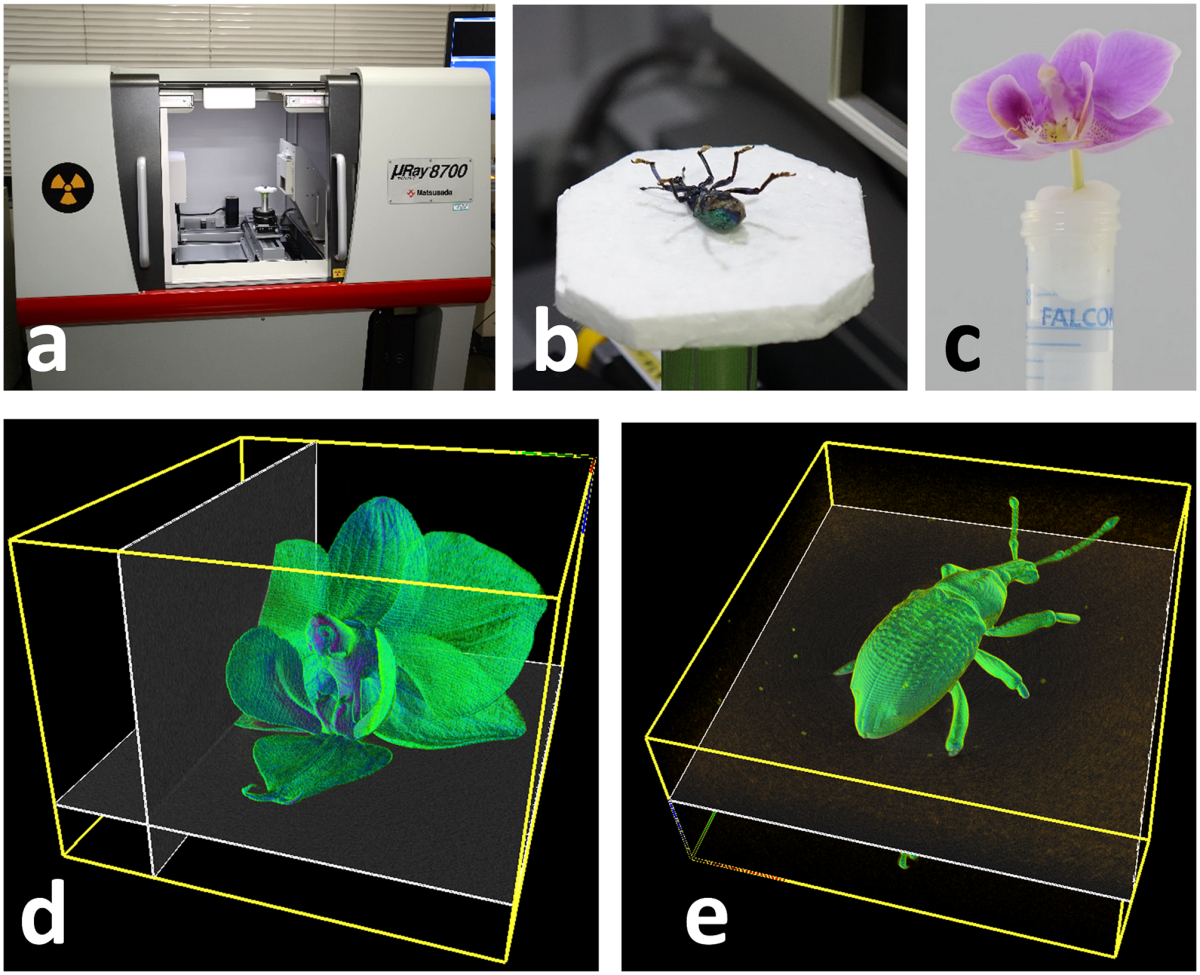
X-ray CT scanning. We used μRay8700 (a). We place insect directly on stage (b) and fix flower by using plastic tube (c). Obtained CT volumes are visualized in (d) and (e).
